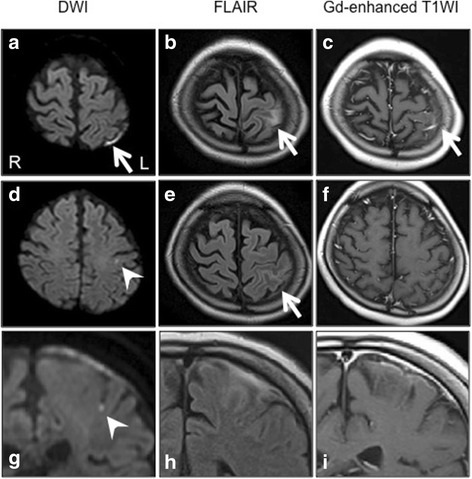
Brain MRI scan findings at admission. a Axial DWI showing restriction of diffusion in the left temporal subarachnoid space (arrow). b, e Axial FLAIR images showing hyperintensity from the same lesion. c, f Axial Gd-enhanced T1-weighted images showing partial enhancement in the left temporal subarachnoid space. d Axial DWI showing a spot lesion indicating restriction of diffusion in the left parietal cortex (arrowhead). g–i Coronal-DWI, FLAIR, and Gd-enhanced T1-weighted views of the same lesion shown in d, e, and f. DWI diffusion-weighted imaging, FLAIR fluid-attenuated inversion recovery, Gd gadolinium
